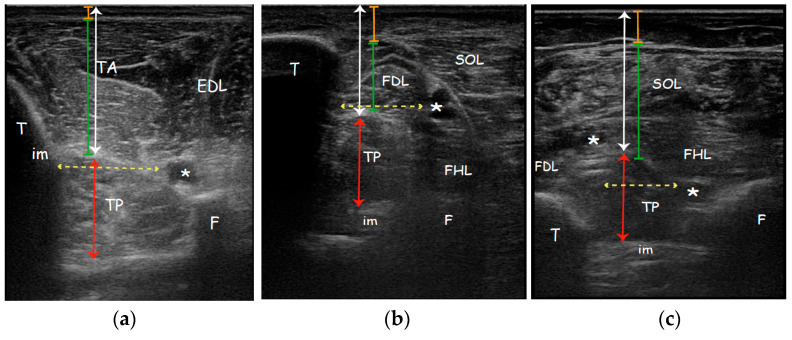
Real ultrasound images of a patient enrolled in the study, affected side. Parameters measured with ultrasonography evaluating the (a) Anterior approach; (b) Medial approach; (c) Posterior approach. Orange line: subcutaneous tissue thickness; Green line: overlying muscle thickness; White arrow: TP muscle depth; Red arrow: TP muscle thickness; Yellow dotted arrow: safety window. Abbreviations: TA tibialis anterior muscle; EDL extensor digitorum longus muscle; TP tibialis posterior muscle; SOL soleus muscle; FDL flexor digitorum longus muscle; FHL flexor hallucis longus muscle; T tibia; F fibula; im interosseous membrane; * neurovascular bundle.
